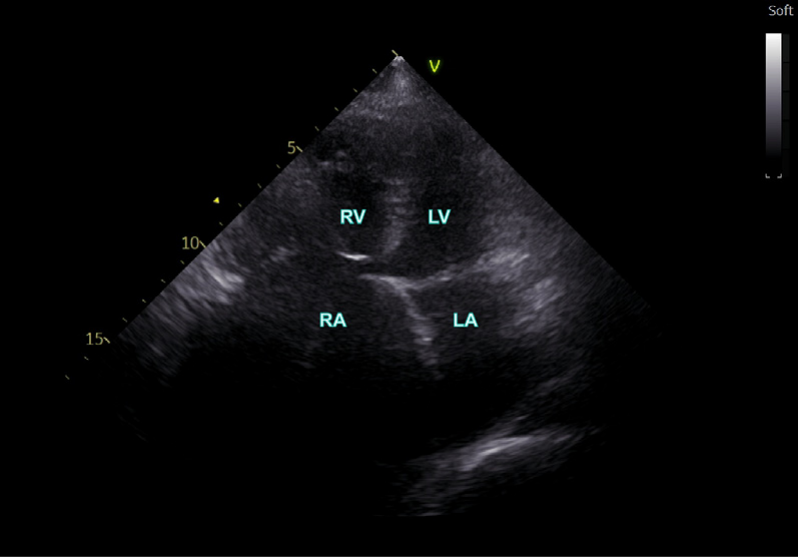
Echocardiogram The echocardiogram demonstrated a significantly enlarged atrium. Specifically, the inner diameter of the left atrium measured 51 mm while that of the right atrium was 71 mm. LA = left atrium; LV = left ventricle; RA = right atrium; RV = right ventricle.
